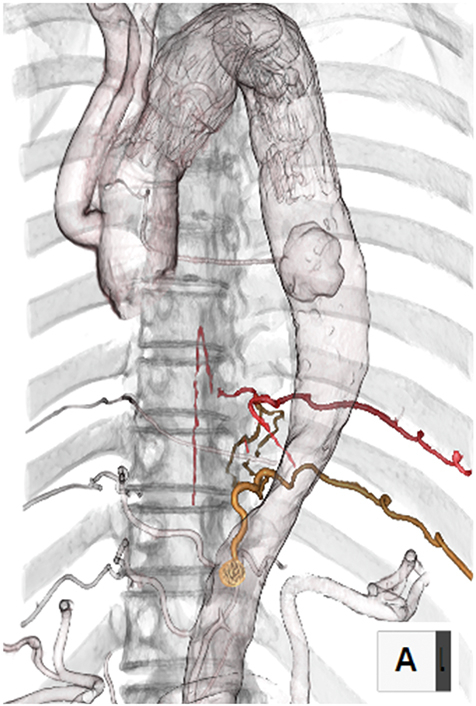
Adamkiewicz artery−specific CTA provided value-added insights over standard CTA.
